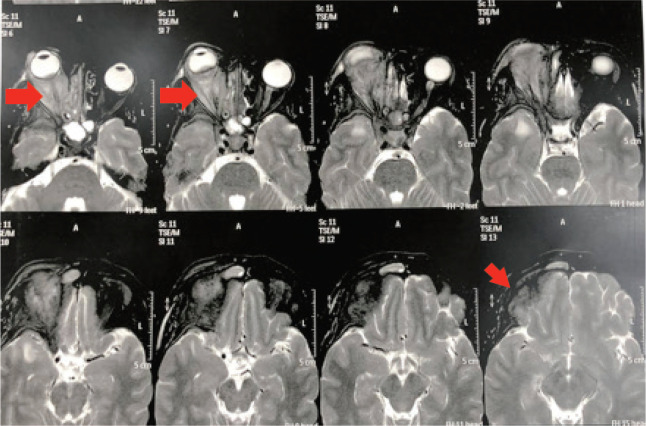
MRI brain and orbit showed significant increase in the previously described right recti muscles thickness with heterogeneous post-contrast enhancement. There is subsequent proptosis and posterior ocular coat mass lesion just above the optic disc with significant enhancement of all posterior ocular coats. There is extra-orbital spread, and intra-cranial extra-axial leptomeningeal enhancement at the right frontotemporal region.
